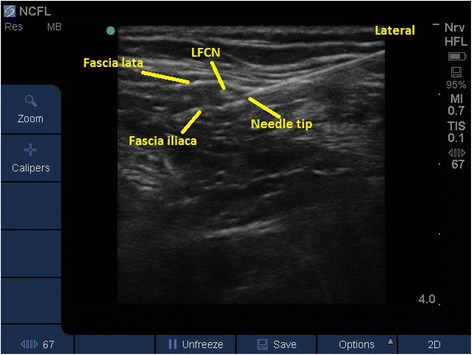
Ultrasound image of the lateral femoral cutaneous nerve. LFCN, Lateral Femoral Cutaneous Nerve
